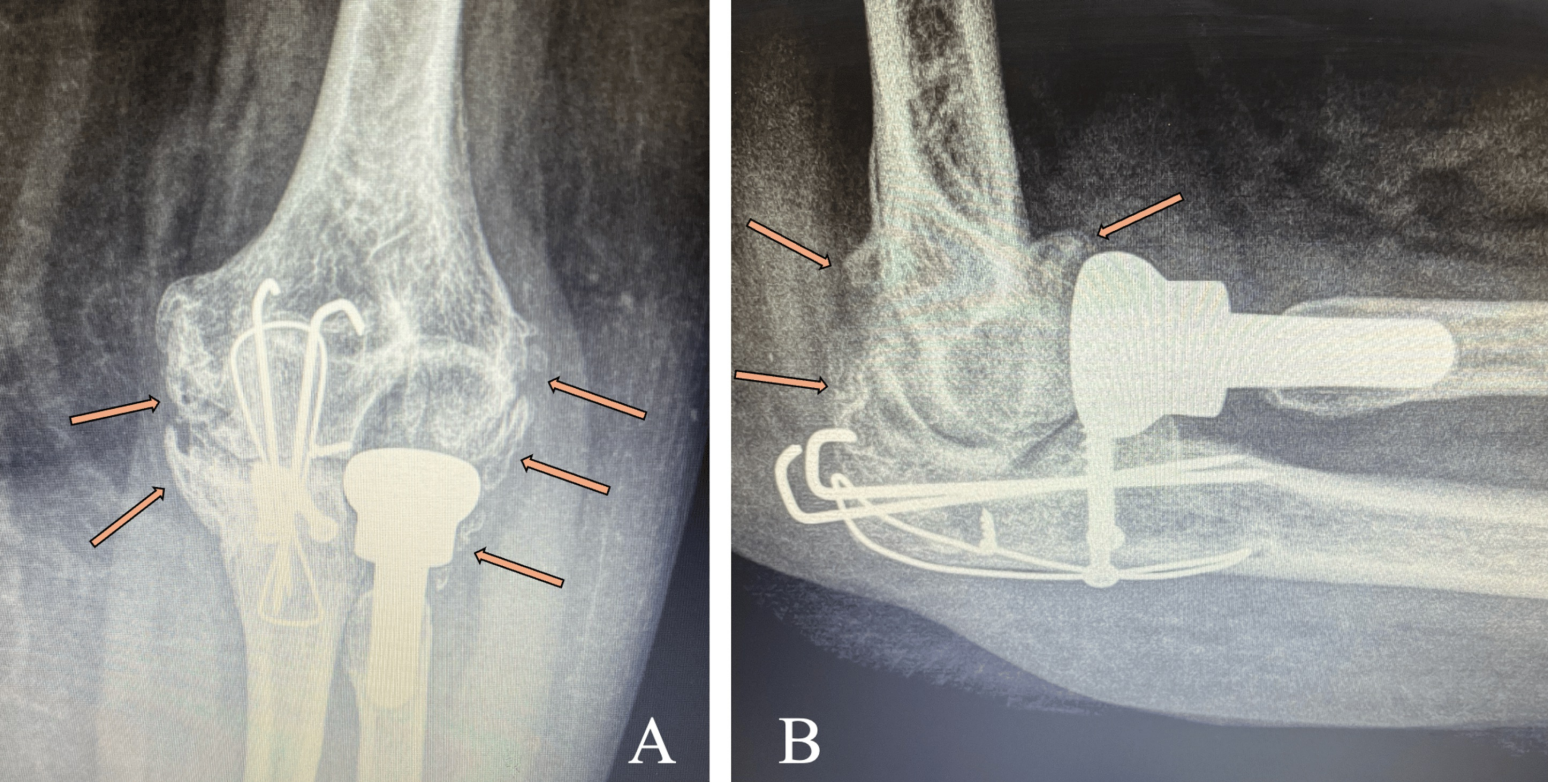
Anteroposterior (A) and lateral (B) radiographs of the left elbow at the final follow-up, demonstrating a well-positioned radial head prosthesis, union of the olecranon fracture, heterotopic ossification (orange arrows), and early-stage post-traumatic degenerative changes.
